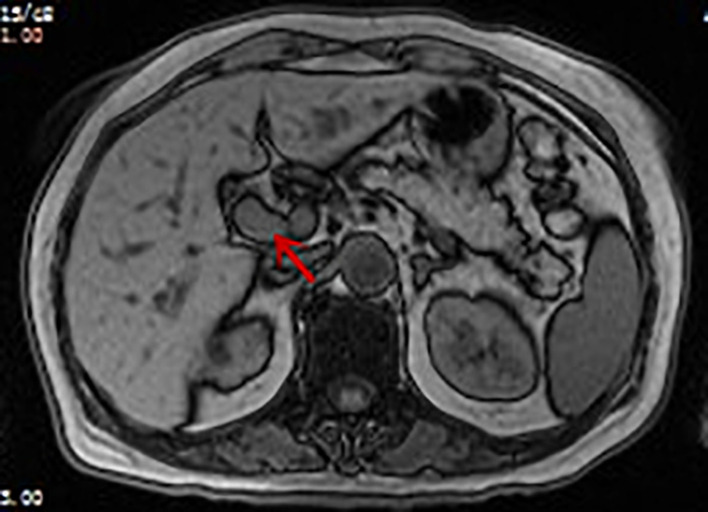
Enhanced MRI image of the patient: soft tissue shadow (indicated by arrow) measuring approximately 1.5 × 1.8 cm can be seen in the hepatic hilum. T1 image: the tumor appears as a slightly low-signal-intensity mass.
